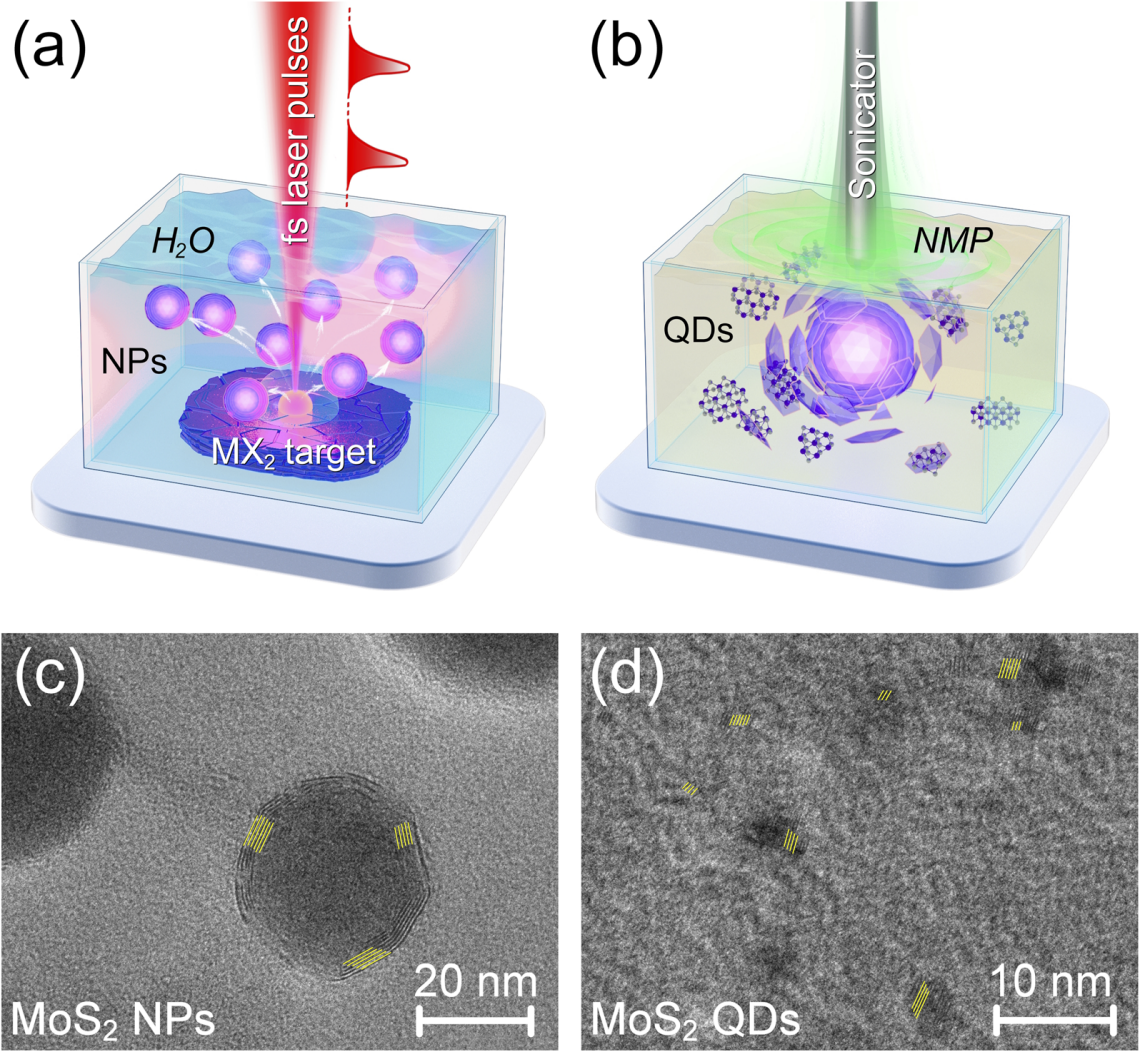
Concept of two-stage synthesis of nanostructures using (a) femtosecond laser ablation of the target placed in water followed by transfer of NPs to NMP and centrifugation for subsequent (b) ultrasonication. TEM images of (c) core–shell NPs synthesized by laser ablation of the MoS_2_ target in water and (d) MoS_2_ QDs obtained after transfer of NPs to NMP and sonication. The average distances between lattice fringes in panels (c) and (d) are 0.61 nm and 0.28 nm, respectively.
